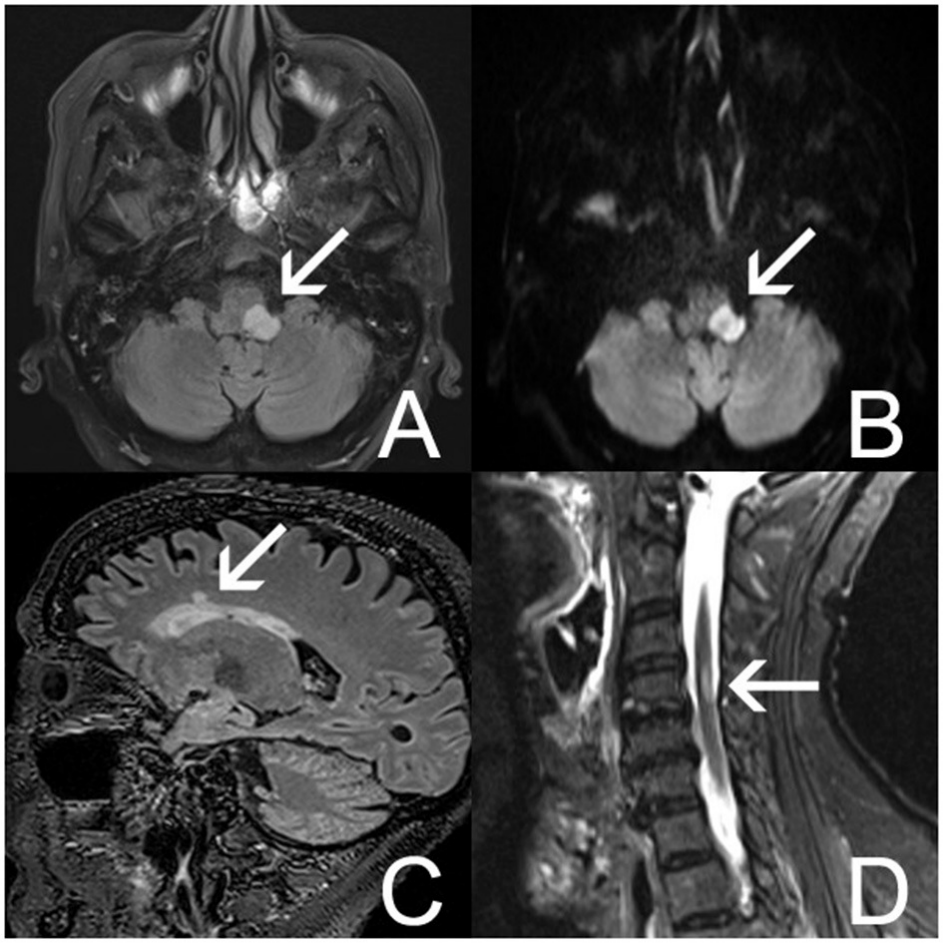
Brain MRI (A–D). (A) Axial fluid-attenuated inversion recovery (FLAIR) MRI image shows a well-demarcated, hyperintense lesion in the left posterolateral part of the medulla oblongata (arrow). (B) Axial diffusion-weighted imaging (DWI) shows restriction of diffusion in this lesion (arrow). (C) Sagittal FLAIR MRI image shows a nodular hyperintensity in the right frontal lobe (arrow). (D) Sagittal short tau inversion recovery (STIR) image shows a hyperintense lesion in the cervical medulla at C4 (arrow).
